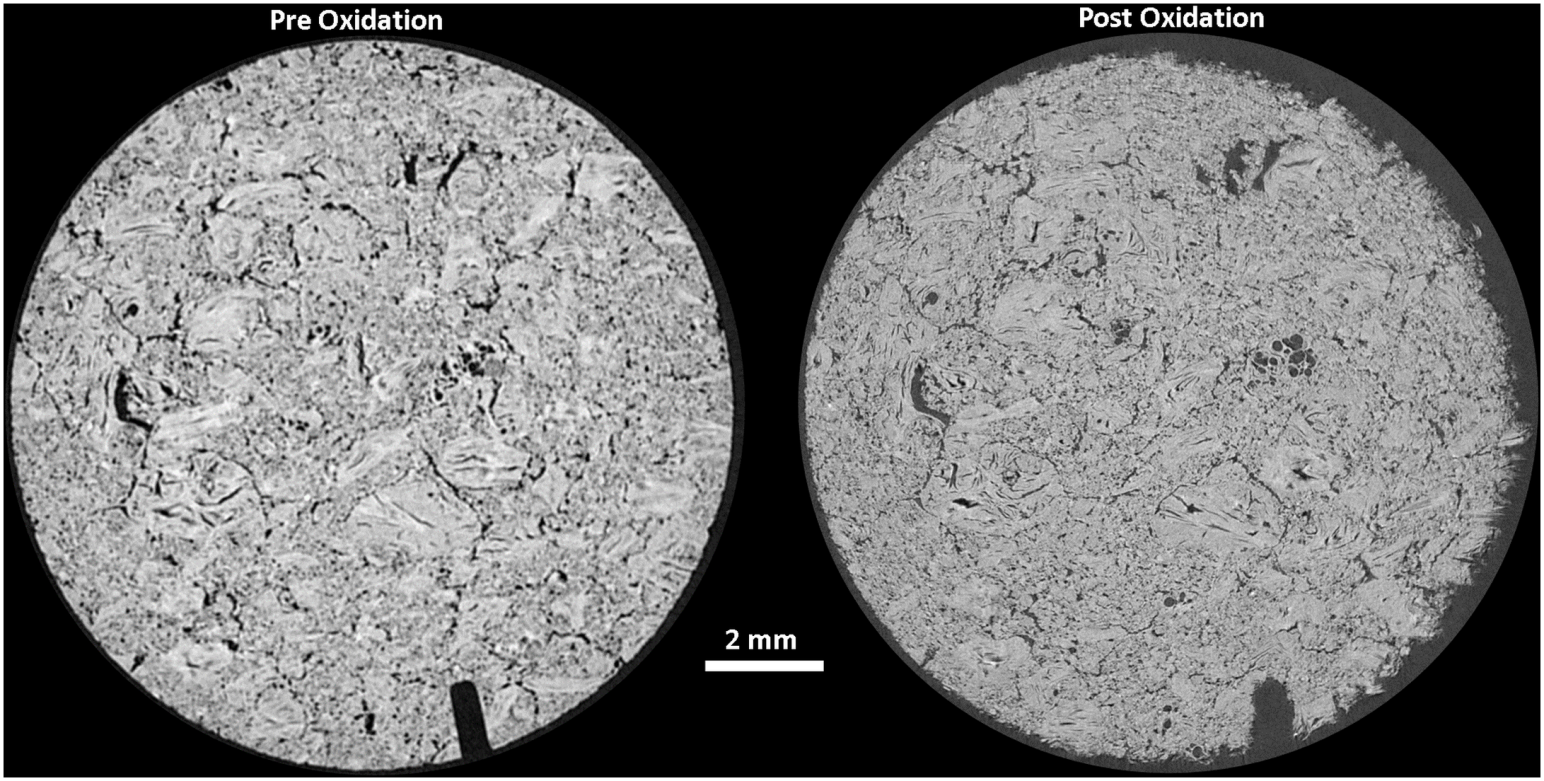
X-ray tomograms from PGA graphite pre and post oxidation at 900°C in air.
